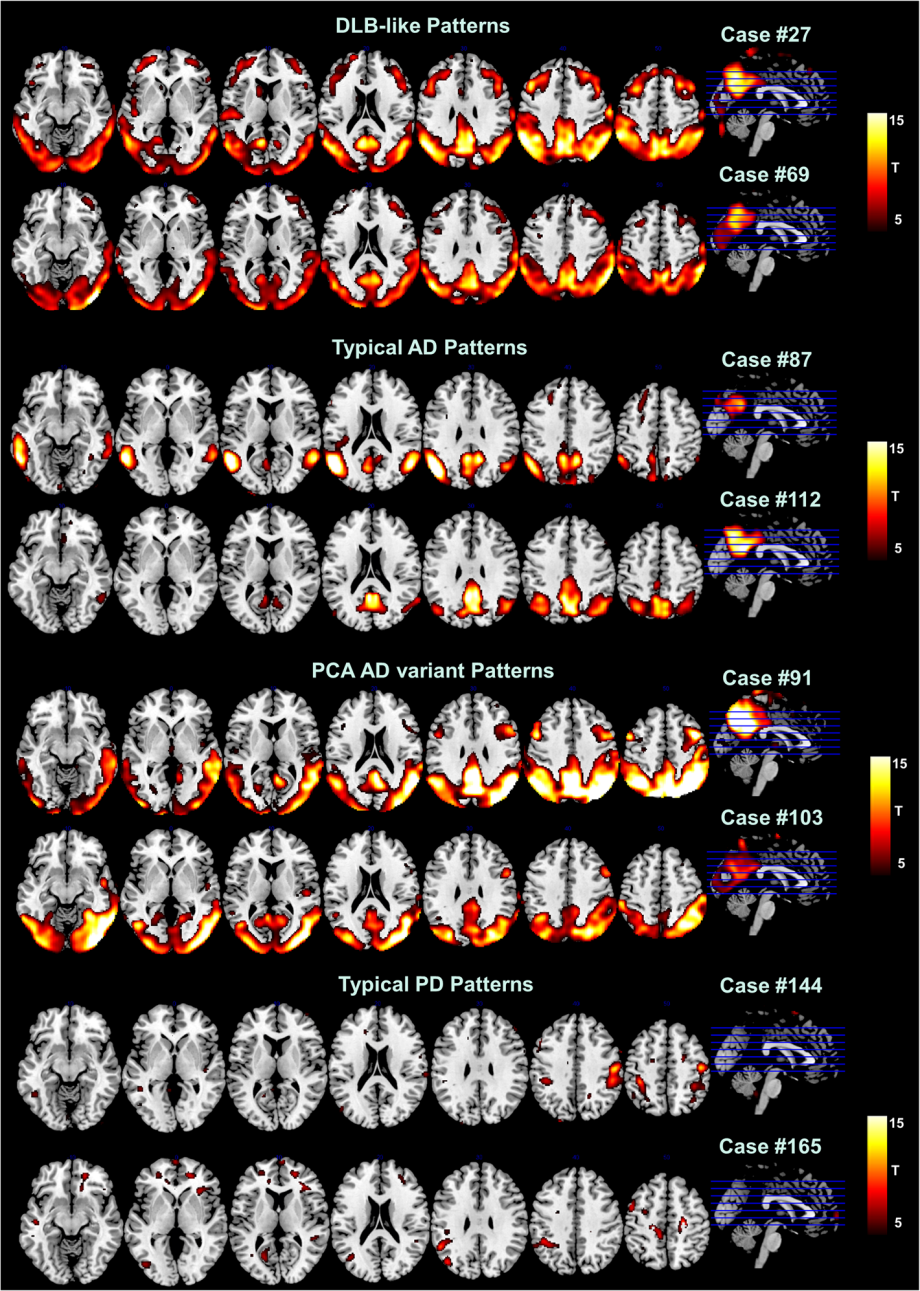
Representative SPM-t-map at the single-subject level. Figure shows examples of [18F]FDG-PET single-subject SPM-t-maps across neurodegenerative conditions. Yellow/red scales represent hypometabolism severity, as obtained from the comparison with the normal control database (*p* < 0.05 FWE-corrected, *k* = 100). Abbreviations: AD, Alzheimer’s disease; DLB, dementia with Lewy bodies; PCA, posterior cortical atrophy; PD, Parkinson’s disease
